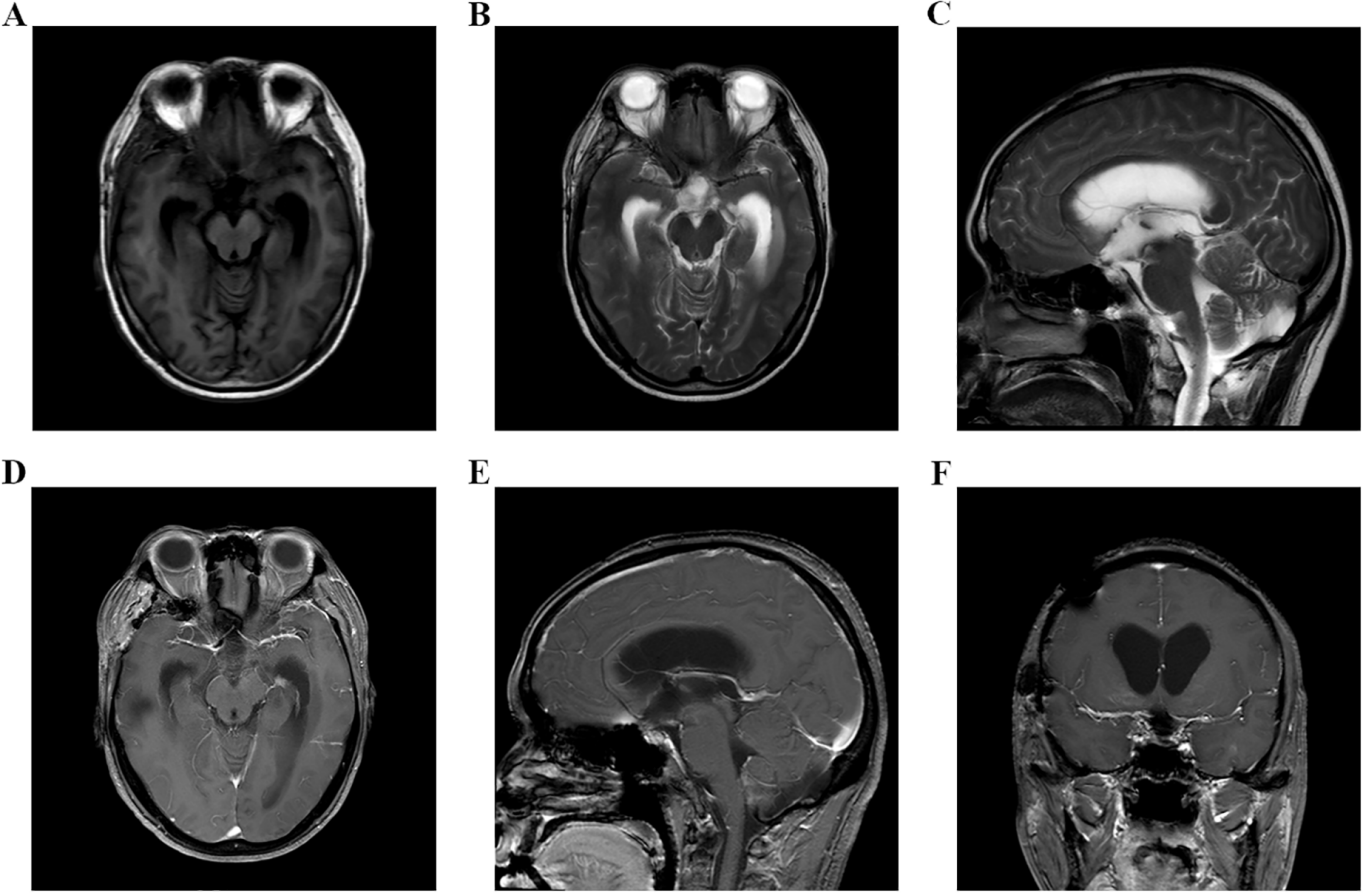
(A–F) Head MRI examination on November 21, 2023, indicated that there was no tumor recurrence in the sellar area, but the third ventricle and bilateral lateral ventricles were dilated.
